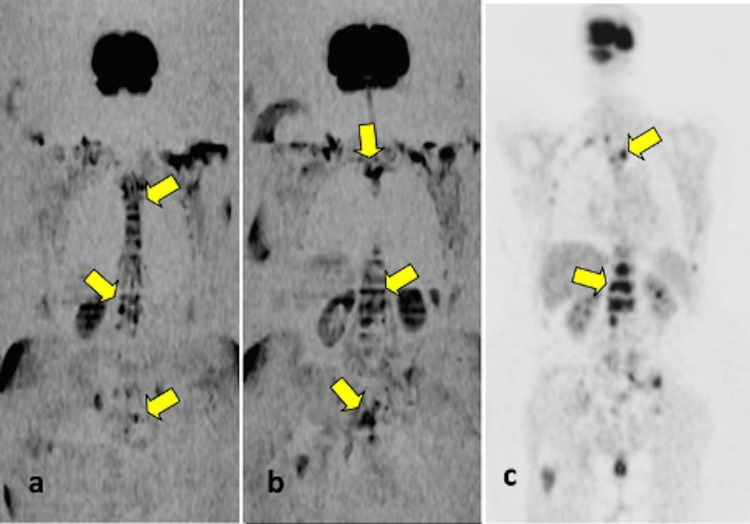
A 62-year-old female patient diagnosed with breast cancer. (a, b) Multiple metastatic signal changes are observed in the vertebrae on coronal inverted whole-body DWI. (c) Coronal PET image shows pathological metabolic activity in the vertebrae. DWI: diffusion-weighted imaging, PET: positron emission tomography.
